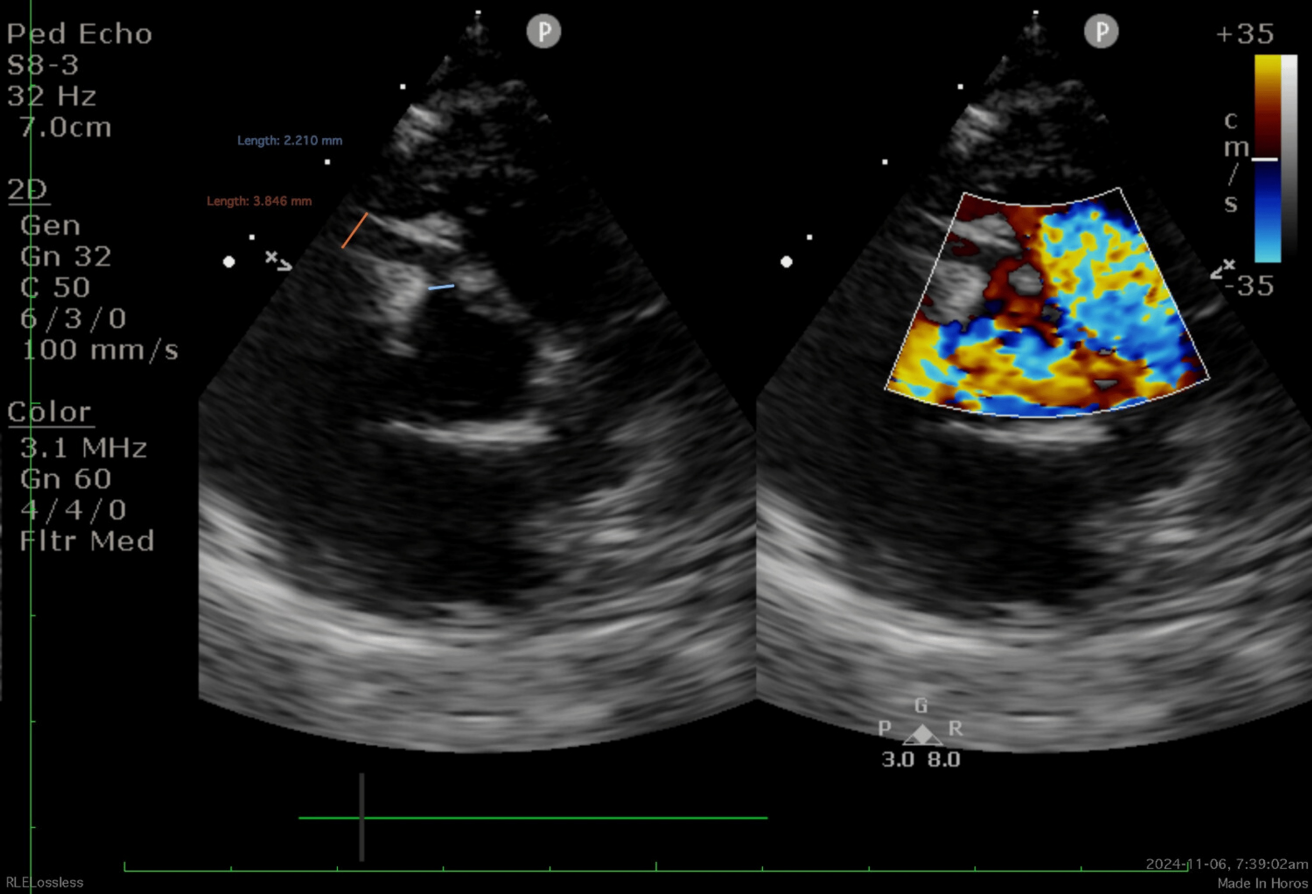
Echocardiography demonstrated proximal right coronary artery (RCA) dilatation measuring 2.2 mm (Z score +2.77) and an RCA aneurysm measuring 3.9 mm (Z score +7.93)
